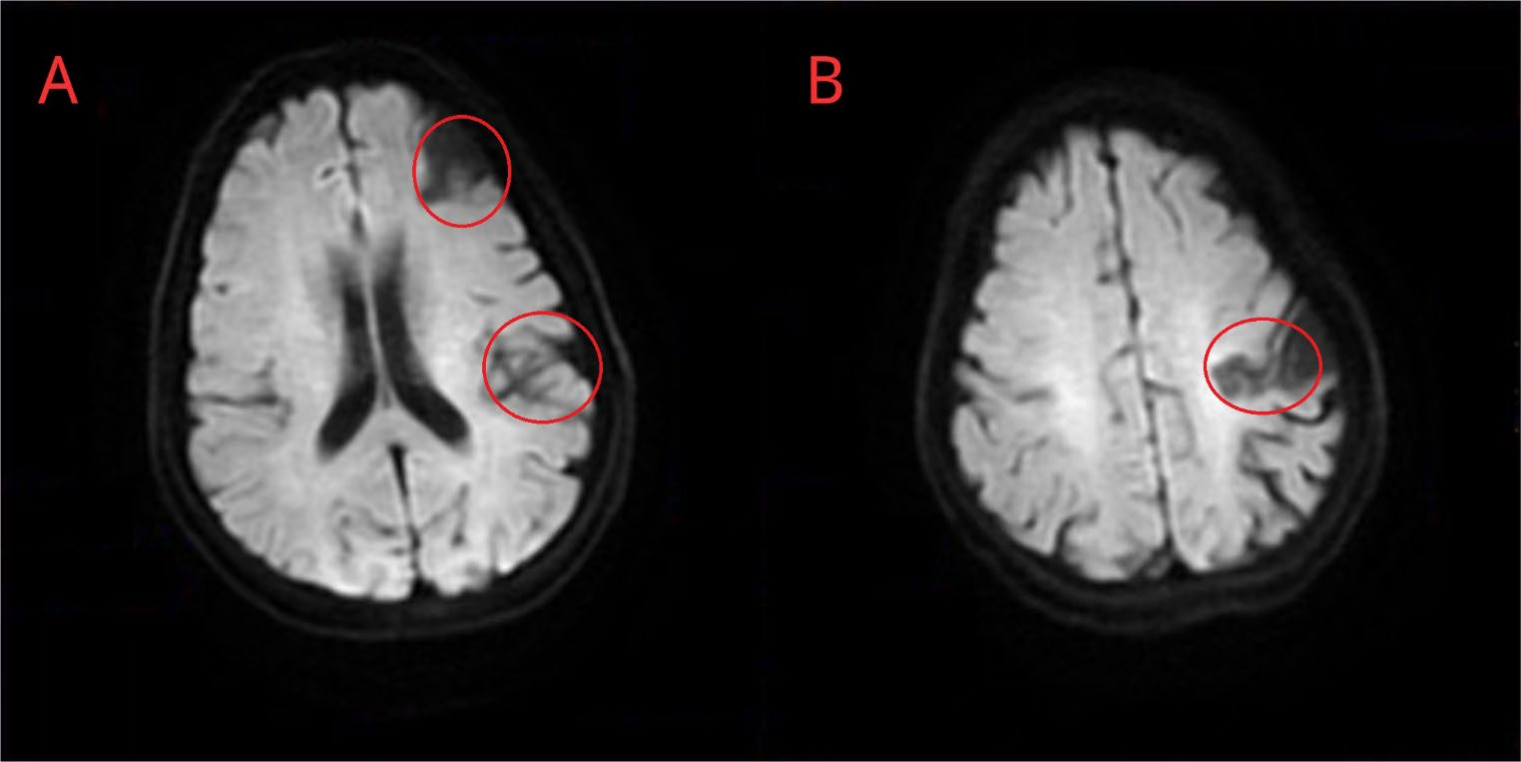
Brain MRI a month after AIS. The MRI reveals brain substance and local fluid signal alterations (red circles) in the left frontal lobe (A) and frontoparietal junction (B), suggesting liquefactive necrosis of the left frontal and frontoparietal infarcts
